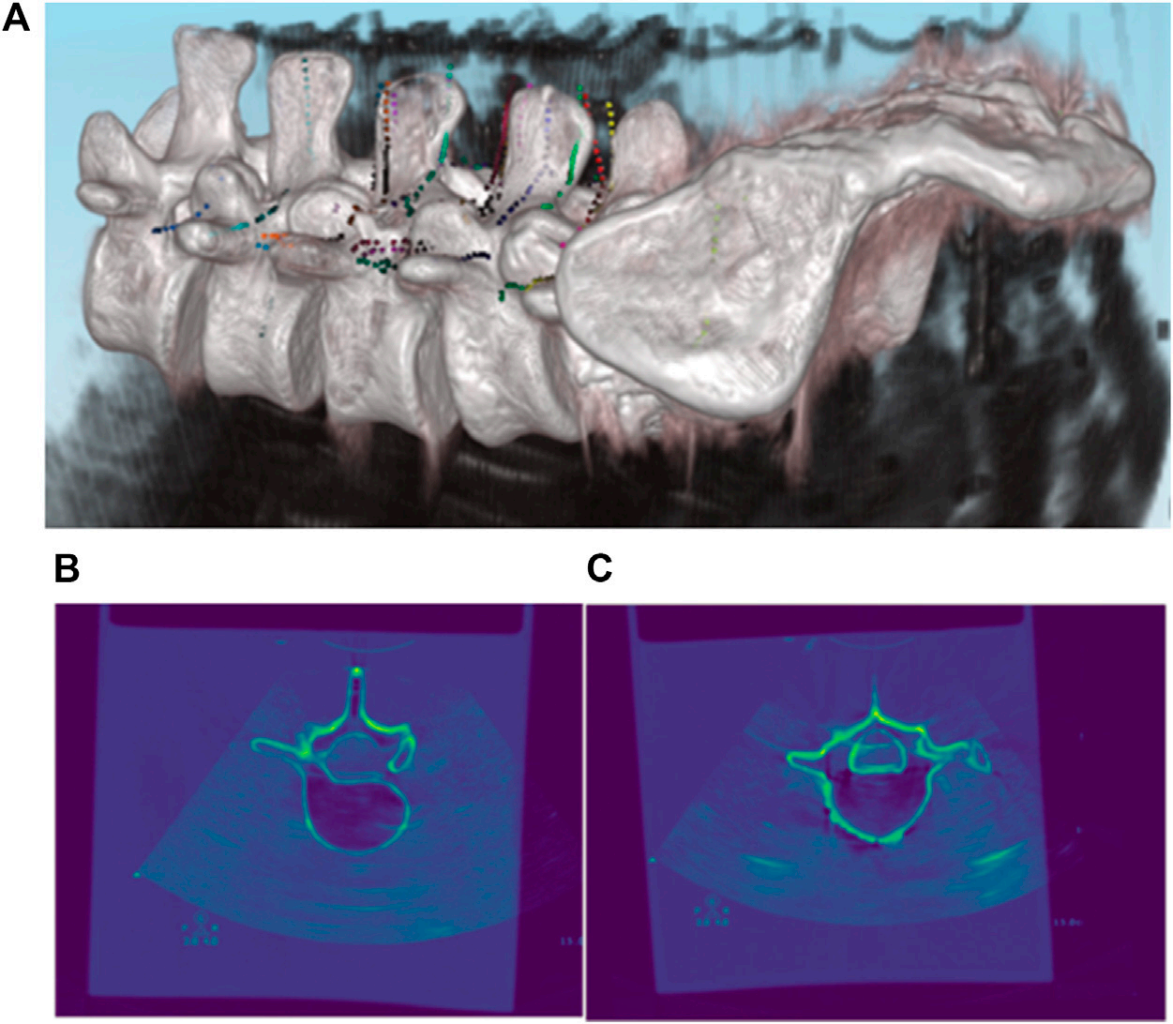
Registration results of pre-operative CT and intra-operative 3D US. (A) 3D Rendering of co-registered CT and US images; (B,C) 2D Rendering of co-registered CT and US images.
